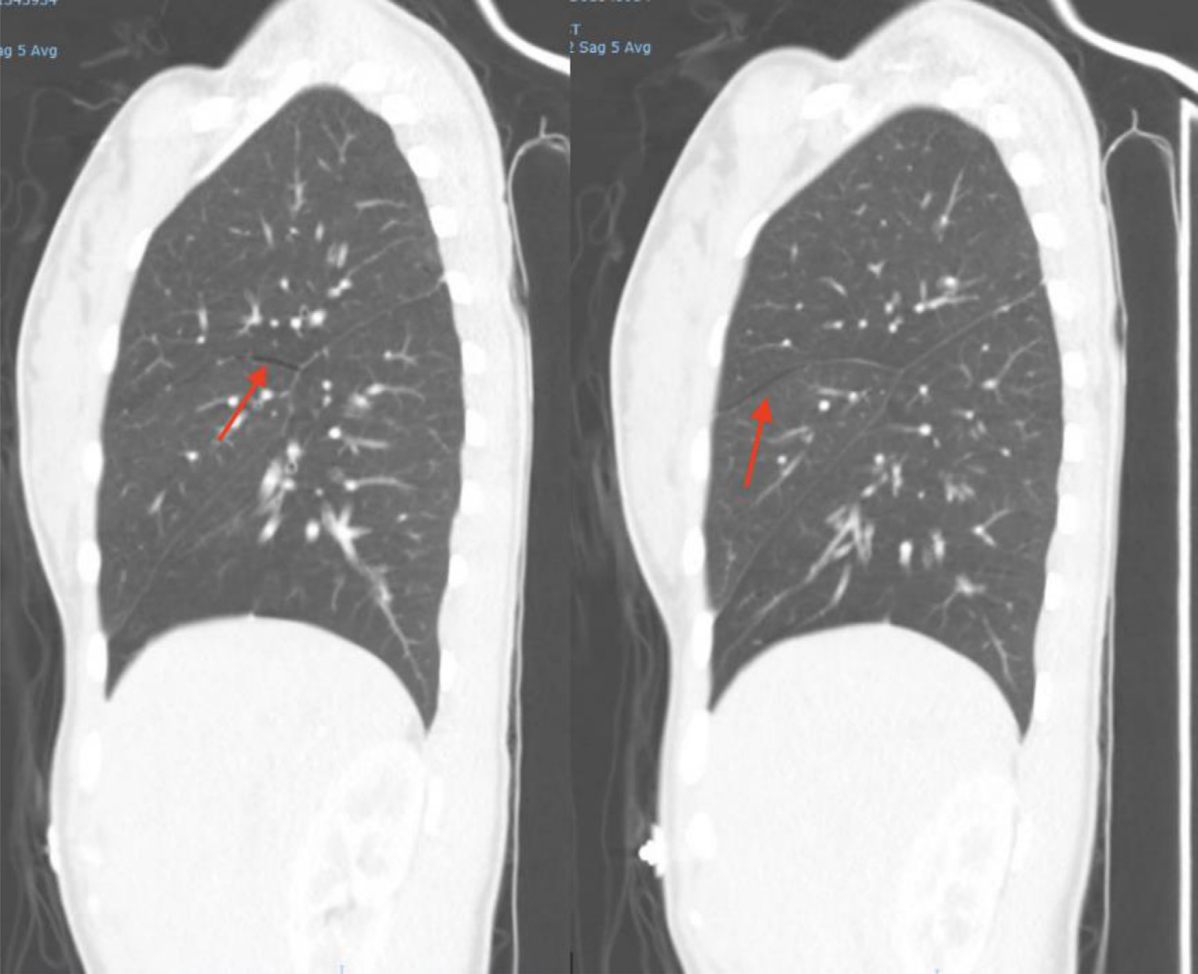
Lateral view of the CT angiogram depicting the hyperlucency of a spontaneous interlobar pneumothorax (red arrow).
